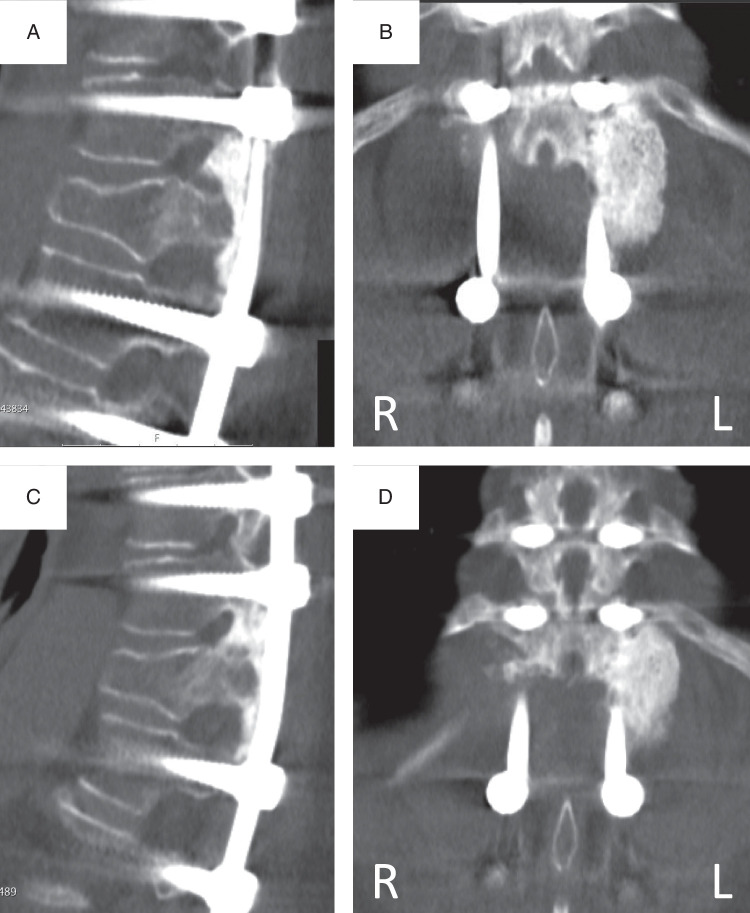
Sagittal and coronal computed tomography images of T12-L1 fusion area with BCP<µm placed on the left side, at 1 year (A and B) and 2 years (C and D) follow-up. One year after surgery the ceramic granules are distinct from bone, fusion was scored as doubtful. After 2 years the granules remodeled to a bony fusion.
